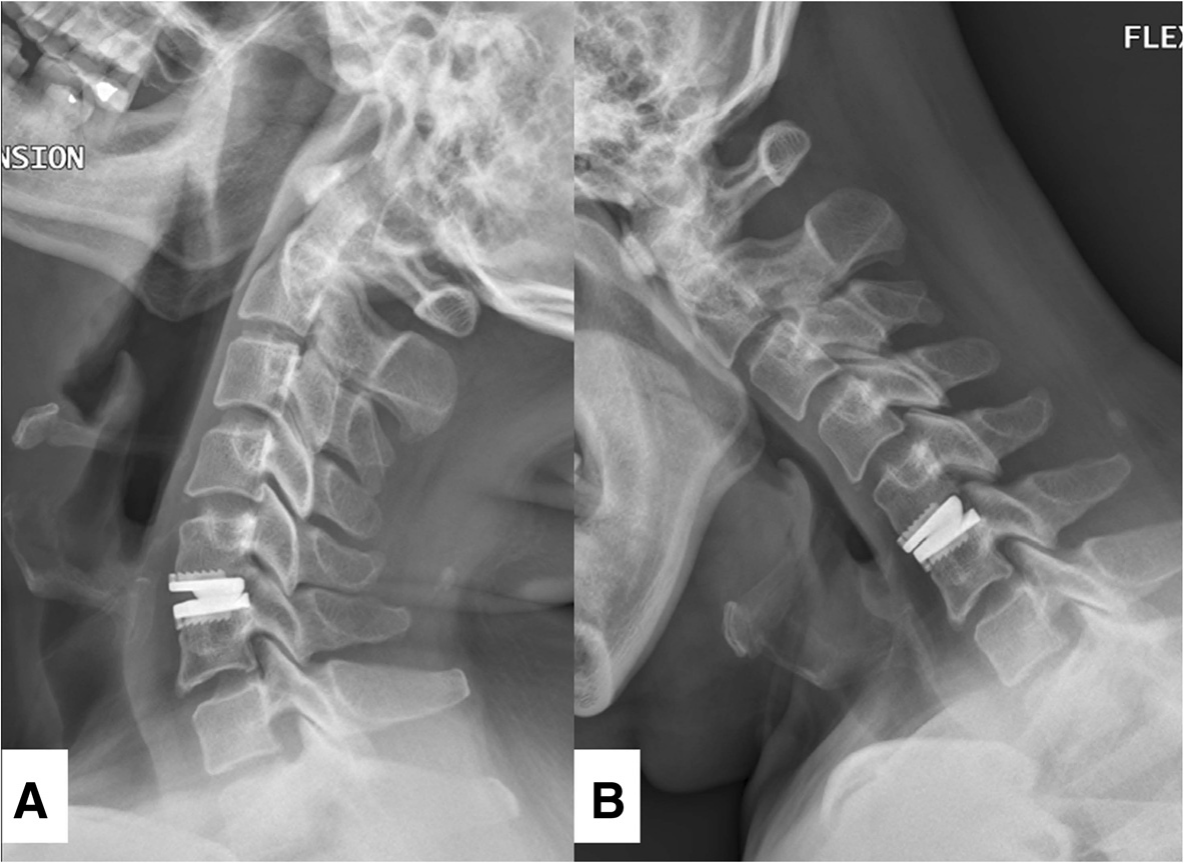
Post-operative dynamic lateral films of the patient mentioned in Fig. 7. The X-ray image demonstrated that the treated levels remained mobile at 24-months follow-up (a: extension, and b: flexion)
